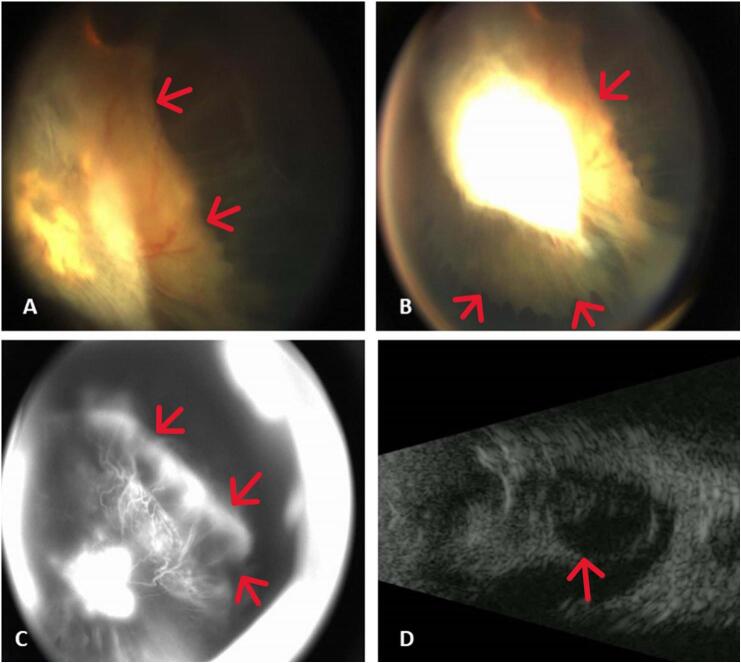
(Right eye). A. Retcam color fundus photo, focused posterior. B. Retcam color fundus photo, focused anterior showing ciliary body traction and funnel retinal detachment. C. Fluorescein angiography, late image. D. B-Scan ultrasound confirming total funnel retinal detachment.
